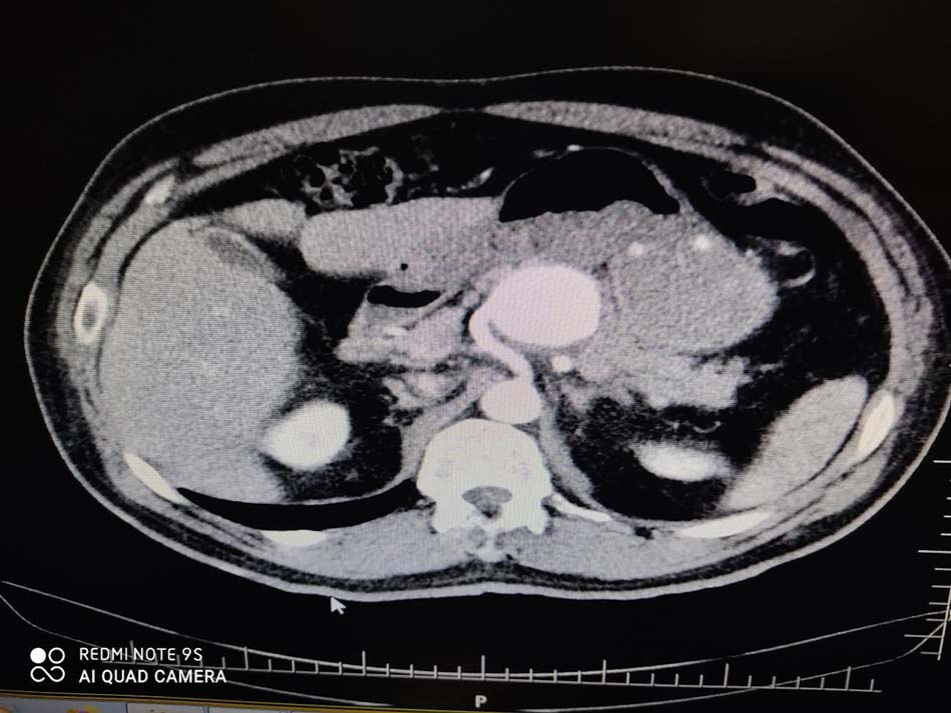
CTA of proximal splenic aneurysm.
